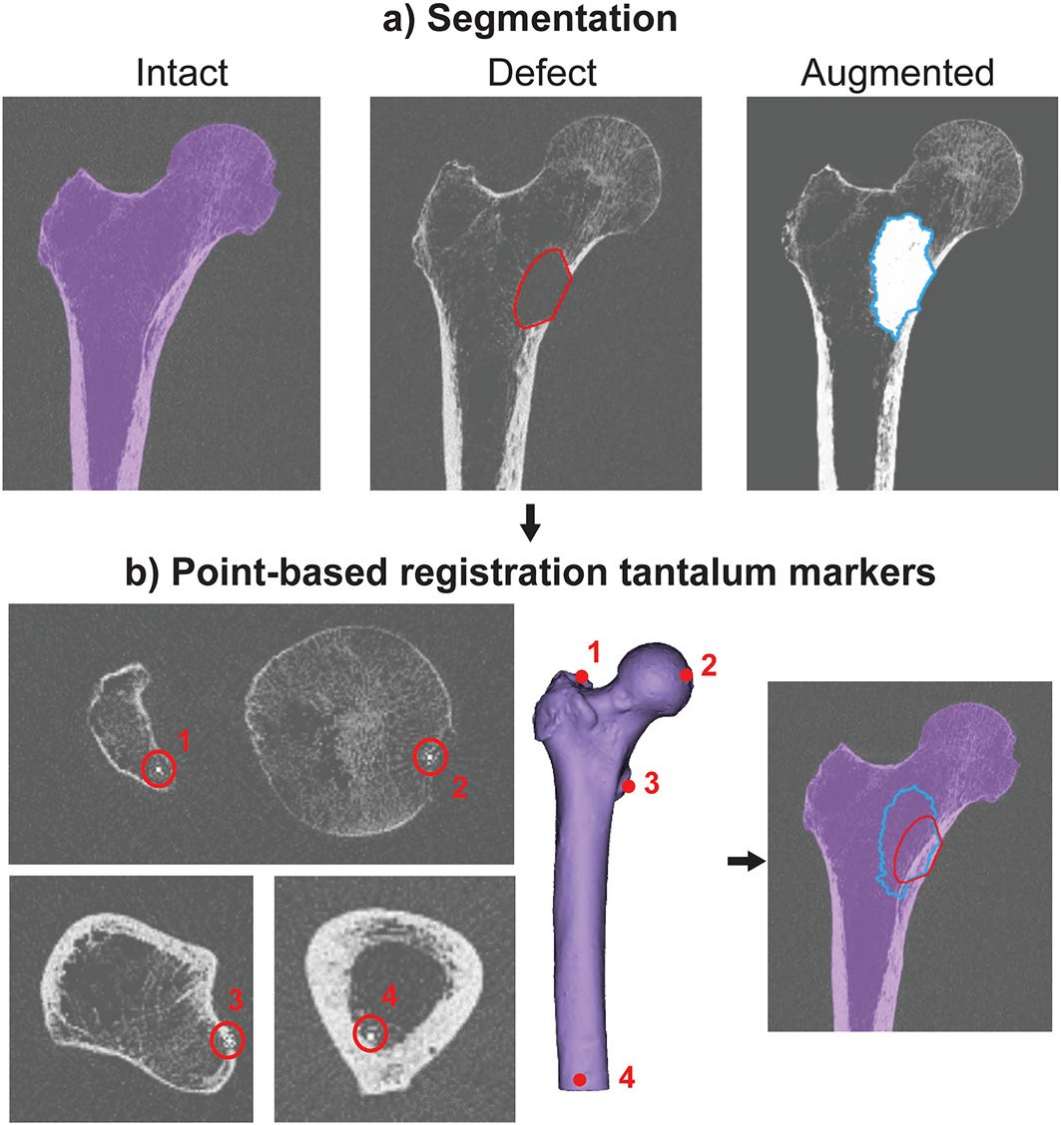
Segmentation and registration. (a) The femur, lesion and cement contour were segmented from the intact, defect and augmented CT scan respectively. (b) The lesion and cement contour were superimposed on the intact CT scan after a point-based registration of the four tantalum markers in each femur [at the top of the greater trochanter (1), the fovea of the femoral head (2), the lesser trochanter (3) and the posterior endosteal surface at the distal end of the femoral shaft (4)].
